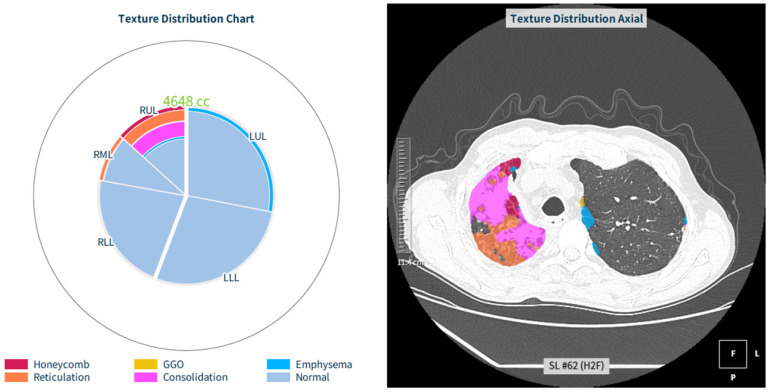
AI-assisted HRCT texture analysis showing regional parenchymal involvement during pulmonary tuberculosis follow-up. The left panel summarizes texture distribution by lobe, and the lower right panel shows the corresponding axial HRCT image with color-coded overlay. The figure highlights the ability of AI-derived outputs to localize residual abnormalities, particularly in the right upper lobe, while reinforcing the need for radiologist verification of software labels in tuberculosis-related cavitary or destructive lesions. Abbreviations: AI, artificial intelligence; HRCT, high-resolution computed tomography; Rt., right; Lt., left; RUL, right upper lobe; RML, right middle lobe; RLL, right lower lobe; LUL, left upper lobe; LLL, left lower lobe; GGO, ground-glass opacity; cc, cubic centimeters.
